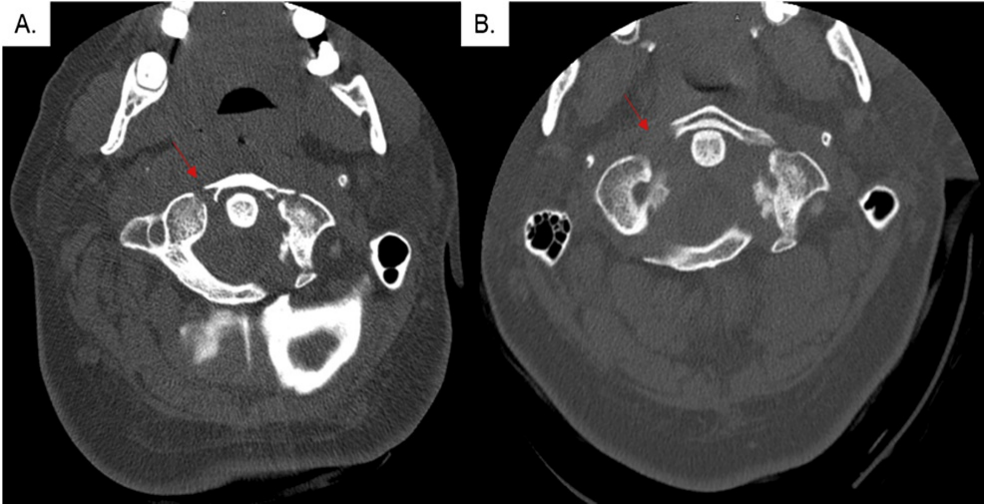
Pre-operative CT of the cervical fracture A) Initial axial non-contrast cervical spine CT centered on the C1 vertebra demonstrates Jefferson fractures involving the anterior (red arrow) and posterior rings, with minimal displacement; B) Follow-up axial non-contrasted cervical spine CT demonstrates worsening displacement and widening of the fracture lines (red arrow).
